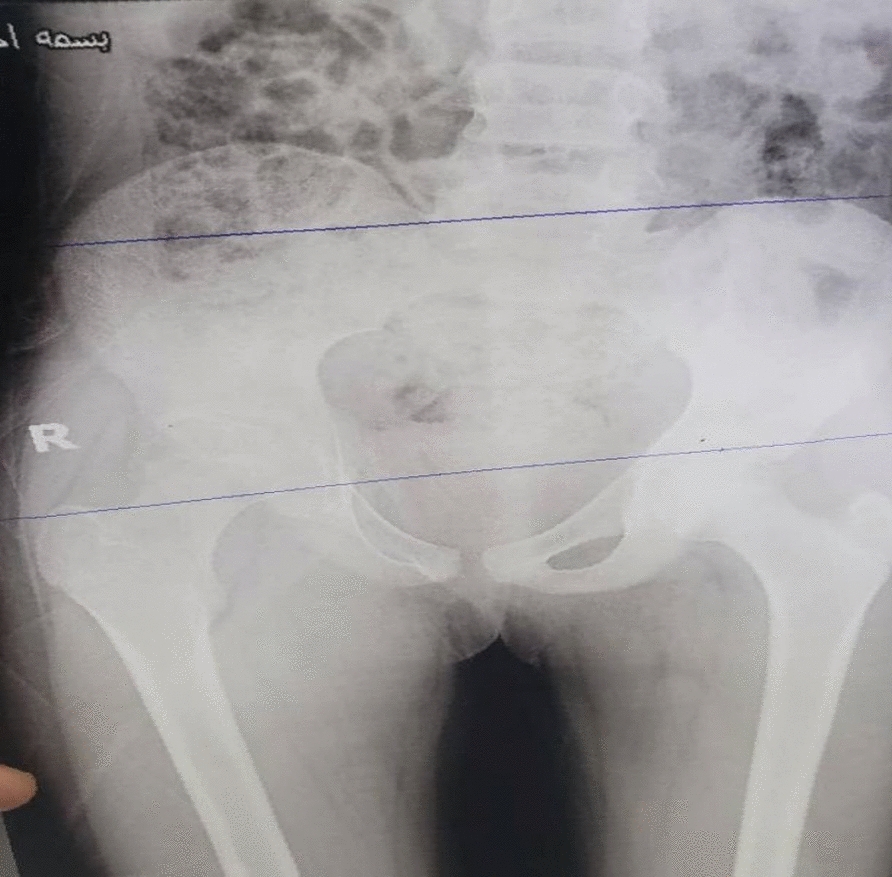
Plain radiographs anteroposterior of the pelvis and hips, which showed narrowing of right joint space with irregularities of femoral head and acetabulum
